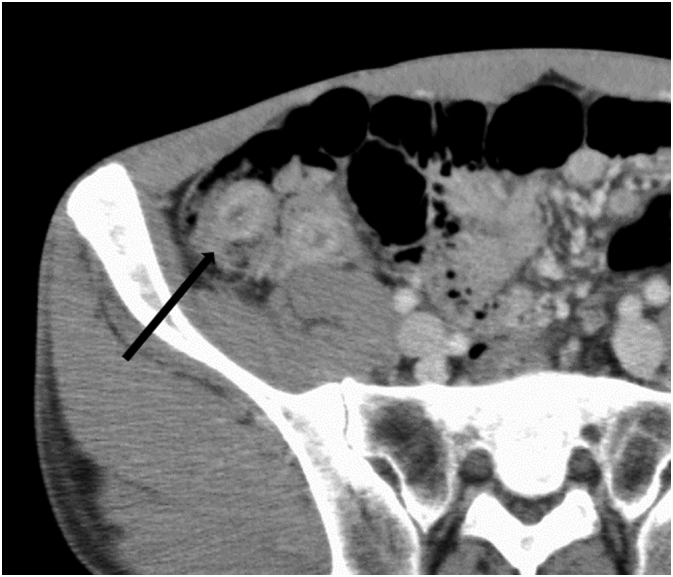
Abdominal enhanced CT showed intussusception of appendix and no tumor on top of it (arrow).
